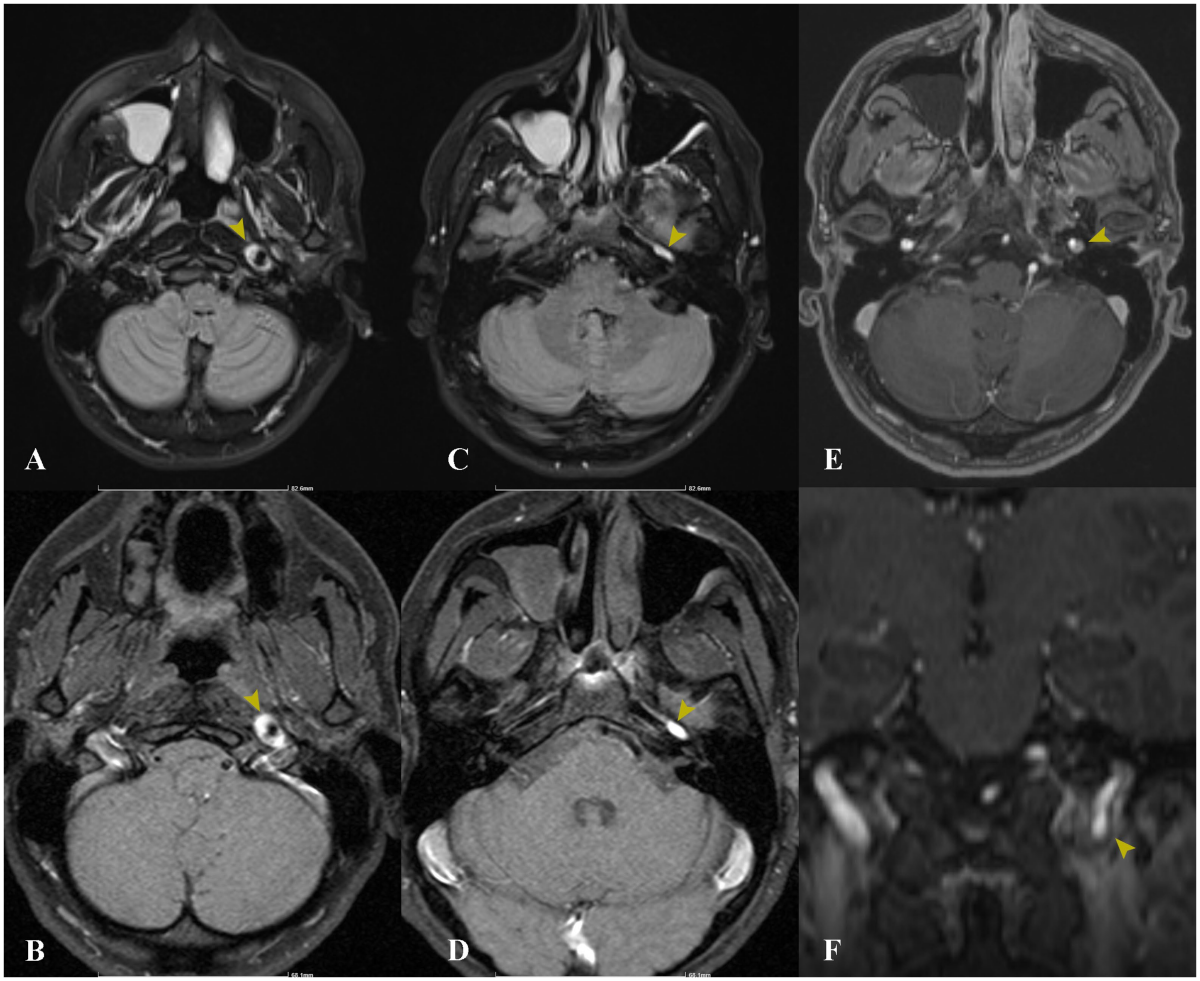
Axial MRI sequences, including FLAIR (A,C) and native T1 (B,D), demonstrate a high signal intensity corresponding to the mural hematoma at the level of the left internal carotid artery (indicated by yellow arrows). Axial (E) and coronal (F) MRI T1 contrast-enhanced sequences reveal the dissection flap and residual lumen (yellow arrows).
